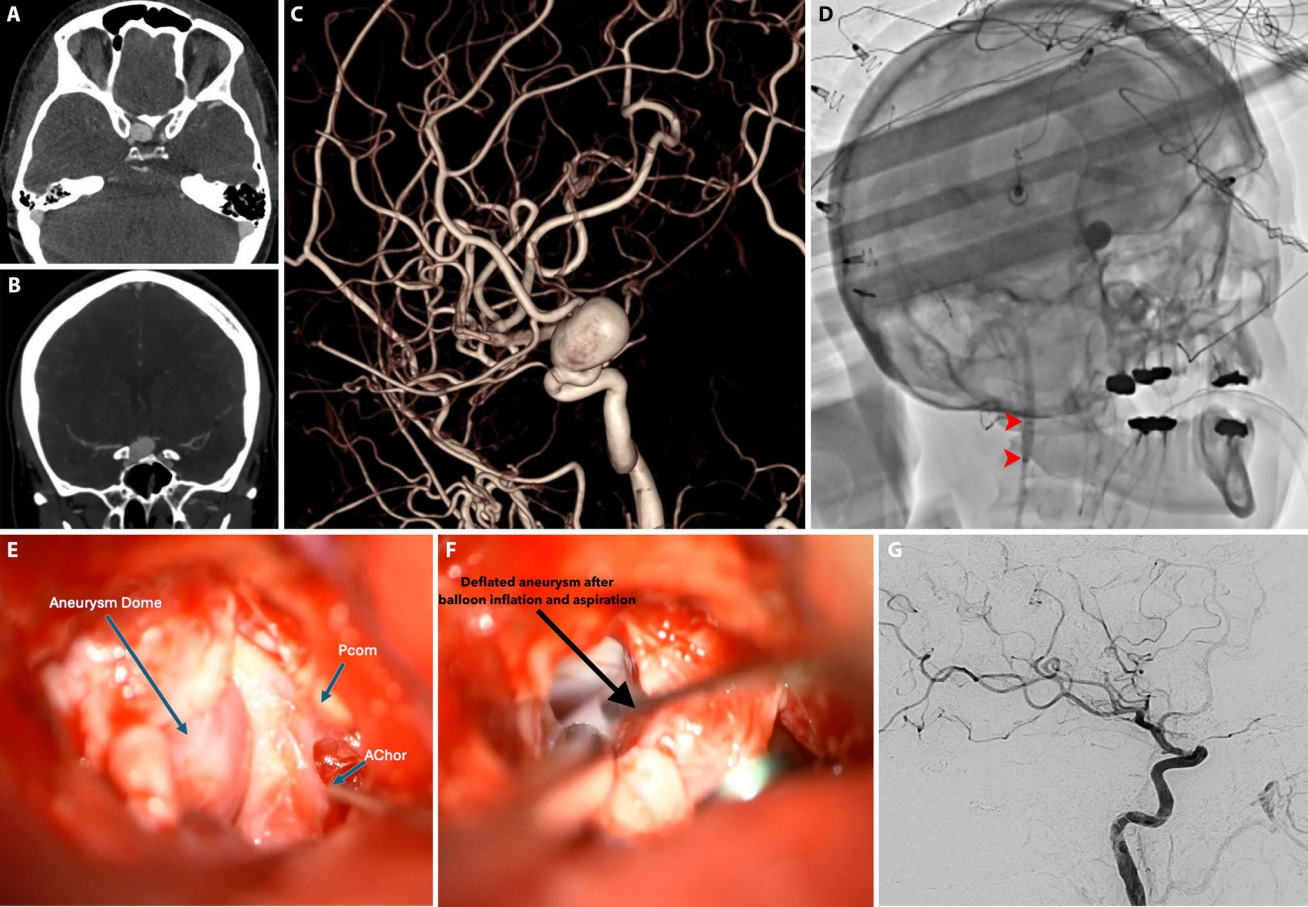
Temporary balloon occlusion (TBO) case example. A Axial and B coronal CT angiogram showing a large, 16 mm right ophthalmic aneurysm originating proximal to the anterior clinoid process. C 3D rotational angiography from a preoperative catheter angiogram right internal carotid artery (ICA) injection showing the large aneurysm in the ophthalmic segment adjacent to the origin of the ophthalmic artery with a 5 mm neck. D Intraoperative unsubtracted single plane lateral angiogram, right ICA injection, at the time of balloon inflation (TBO) in the cervical segment of the ICA showing contrast stagnation distal to the balloon (marked proximally and distally by the red arrowheads) and in the aneurysm. E Intraoperative exposure after a right pterional craniotomy, intradural anterior clinoidectomy. The origins of the posterior communicating artery (Pcom) and anterior choroidal artery are visible lateral to the large aneurysm dome. F Intraoperative view after further dissection and opening of the of the distal dural ring, placement of a temporary clip on the ICA distal to the aneurysm but just proximal to the Pcom, and after inflation of the endovascular balloon (as shown in D) with aspiration decompression. The aneurysm has visibly lost its turgor and can be more easily manipulated with microdissectors for precise clip placement. G Post-clipping intraoperative lateral angiogram (right ICA injection) demonstrates occlusion of the paraophthalmic aneurysm with persistent patency of the nearby ophthalmic artery
